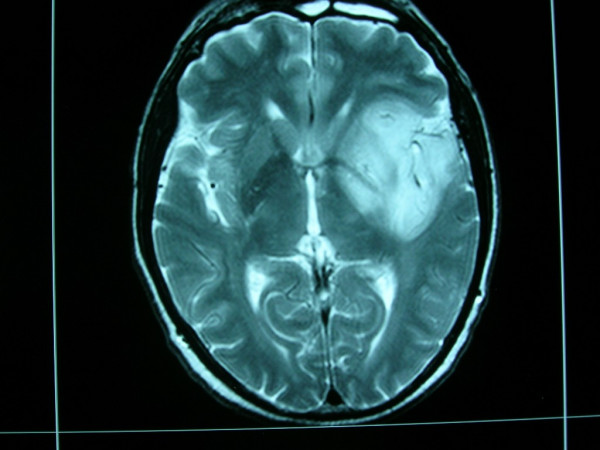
Brain magnetic resonance imaging after the onset of seizures.
